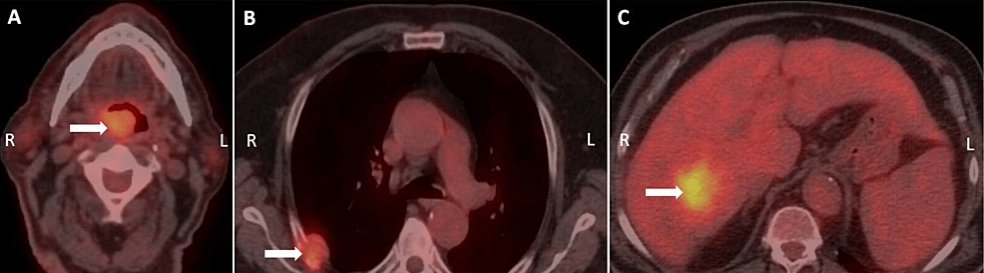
18F-FDG PET/CT. (A) A lesion in the right tonsil (arrow) with intense hypermetabolic activity, (B) a lytic lesion on the right fifth rib (arrow) with an accompanying intensely hypermetabolic soft tissue mass, and (C) a mass in segment VII of the liver (arrow) with an intense hypermetabolic activity. Higher SUVmax was recorded for the liver mass.
